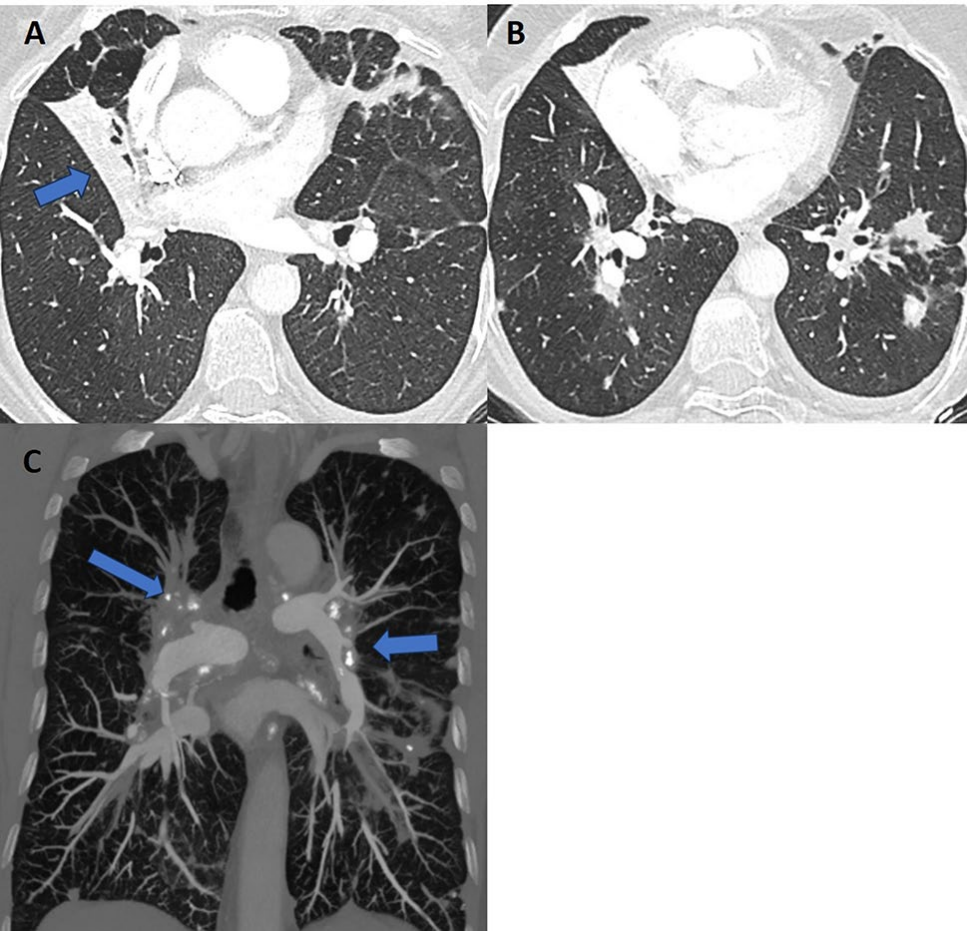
Computed tomography of the chest at the time of pulmonary hypertension diagnosis: (a) and (b) axial images show irregular diffuse nodular and micronodular lesions forming conglomerates and causing bronchial stenosis, atelectasis of the middle lobe (blue arrow) with no endobronchial lesions and impaired ventilatory pattern of the lingula, areas of ground glass and air trapping; (c) coronal MIP reconstruction reveals multiple slightly enlarged hilar and bronchopulmonary lymph nodes with calcifications (blue arrows)
